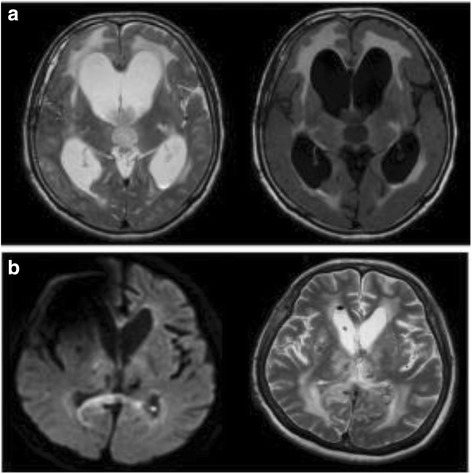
a Magnetic resonance imaging on the 25th day of admission shows abnormal hyperintensities in the trigones of both lateral ventricles, suggesting debris (*left panel*). The central panel and fluid-attenuated inversion recovery imaging (*right panel*) show abnormal periventricular intensities. b During discharge magnetic resonance imaging. Both ventriculitis and hydrocephalus have been resolved
